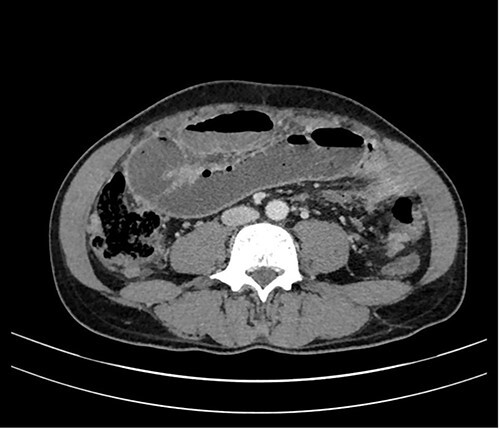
CT axial view: peritoneal thickening and mechanical ileus.
